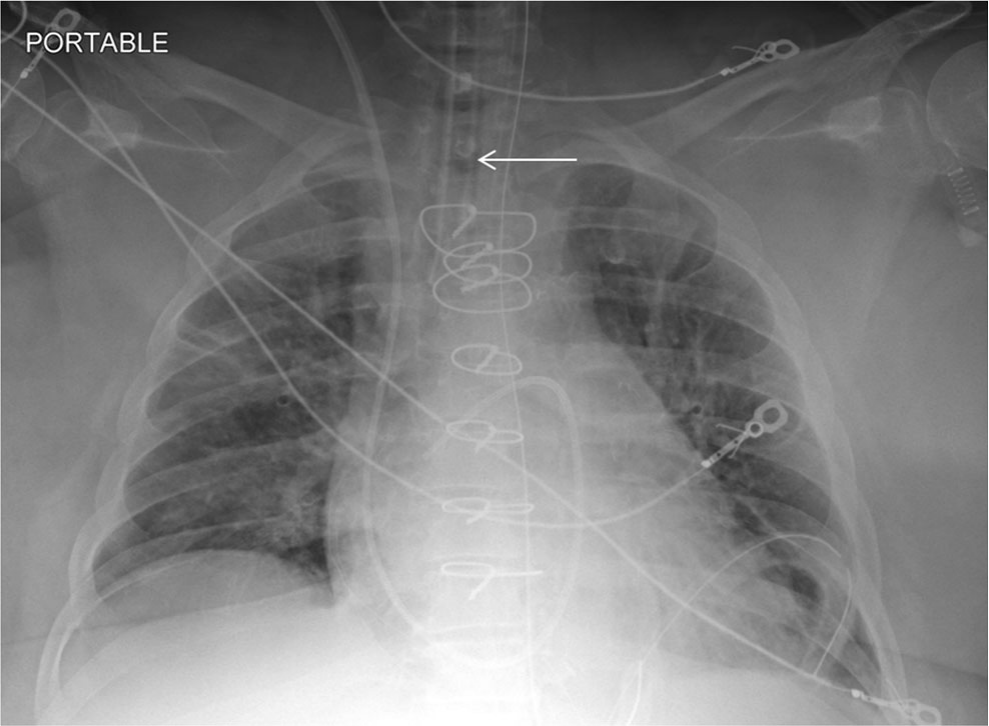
Chest radiograph with ET tube present (*white arrow*)
